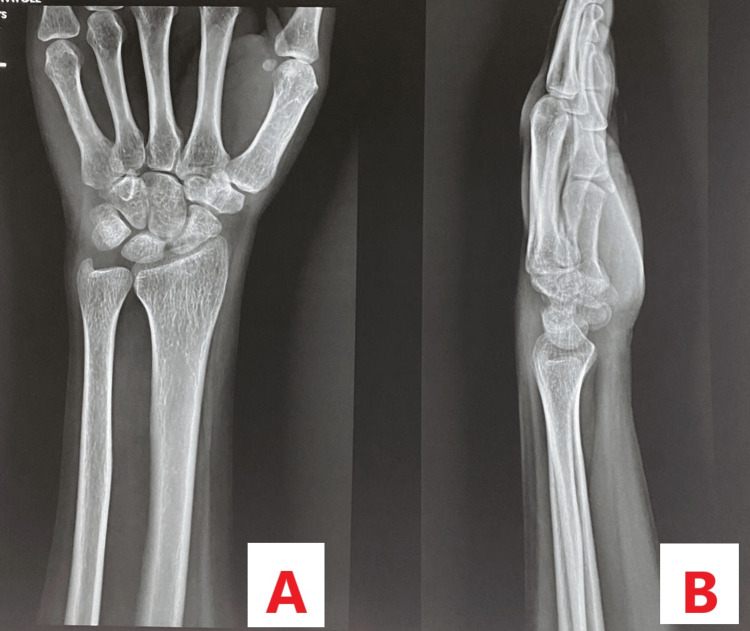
Plain radiograph of the left wrist joint showing no abnormality. A: Plain radiograph showing the posteroanterior view. B: Plain radiograph showing the lateral view.
